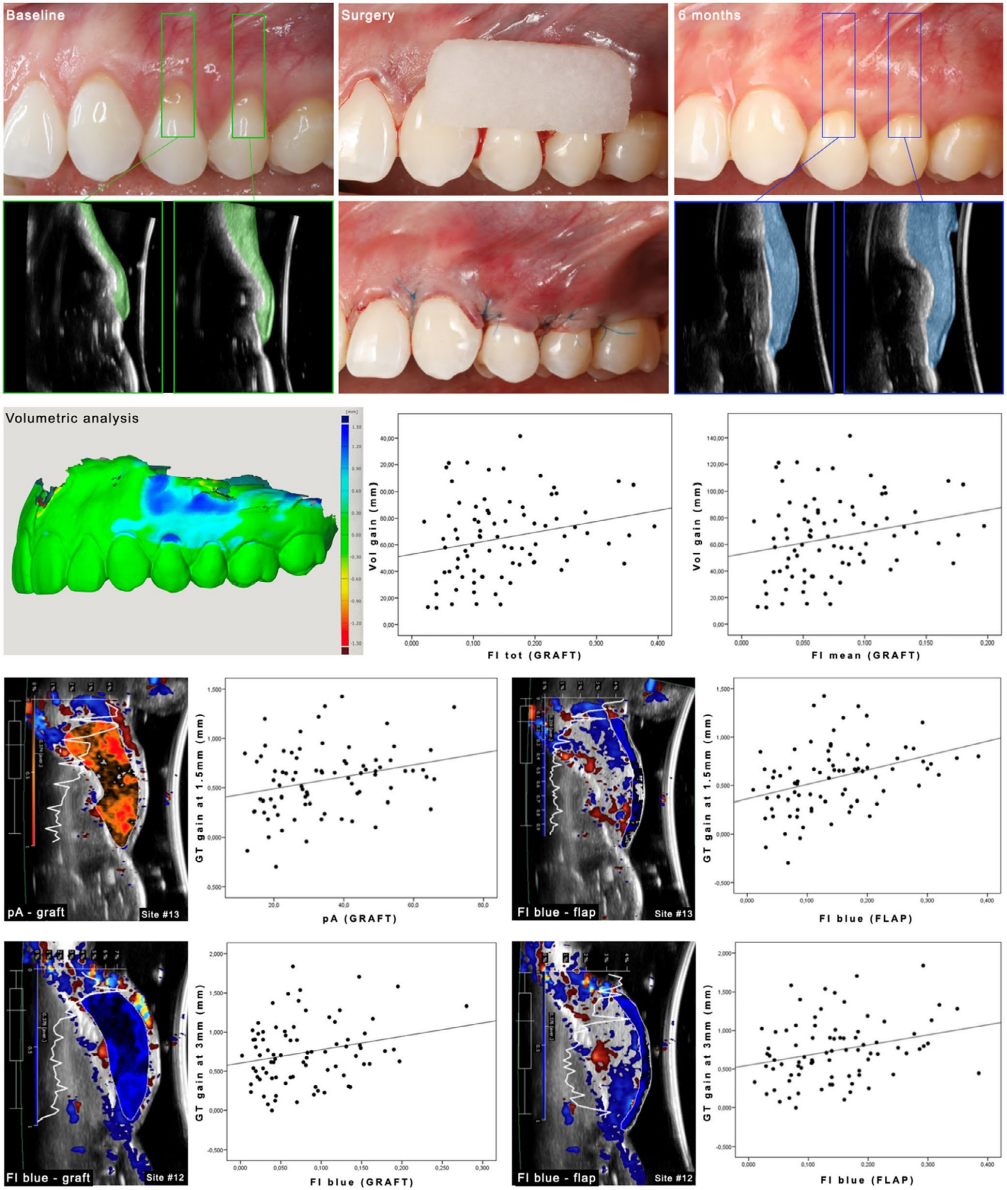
The upper panel shows the baseline clinical and ultrasonographic views as well as the intrasurgical photos and the outcomes at 6 months. The soft tissue of the two premolars at baseline was highlighted in green, while the augmented soft tissue at 6 months was depicted in light blue. The respective volumetric analysis obtained from the superimposition of the digital models at baseline and 6 months is reported below, together with the plot depicting the correlation between FI_tot_, FI_mean_, and final volume gain (Vol gain). The lower panels exhibit the assessment of pA and FI_blue_ at the level of the graft or the flap, with the respective plot highlighting the correlations of these parameters with gingival thickness (GT) gain at 6 months.
